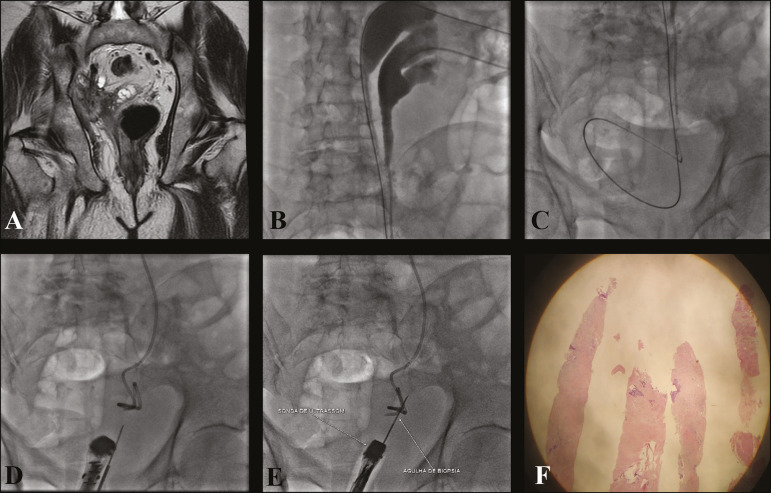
A: T1-weighted magnetic resonance imaging of the pelvis showing marked hydronephrosis of the right kidney, along with an extraperitoneal pelvic lesion in the distal ureter and signs of invasion of the bladder, seminal vesicle, and prostate. B: With the patient in the supine position, pyelography was performed before the procedure that confirmed complete ureteral duplication. C: Percutaneous insertion of a double J catheter (upper cup) and nephrostomy (lower cup). D,E: Endorectal ultrasound and fluoroscopy-guided percutaneous biopsy. F: Anatomopathological report revealed poorly differentiated urothelial neoplasia.
